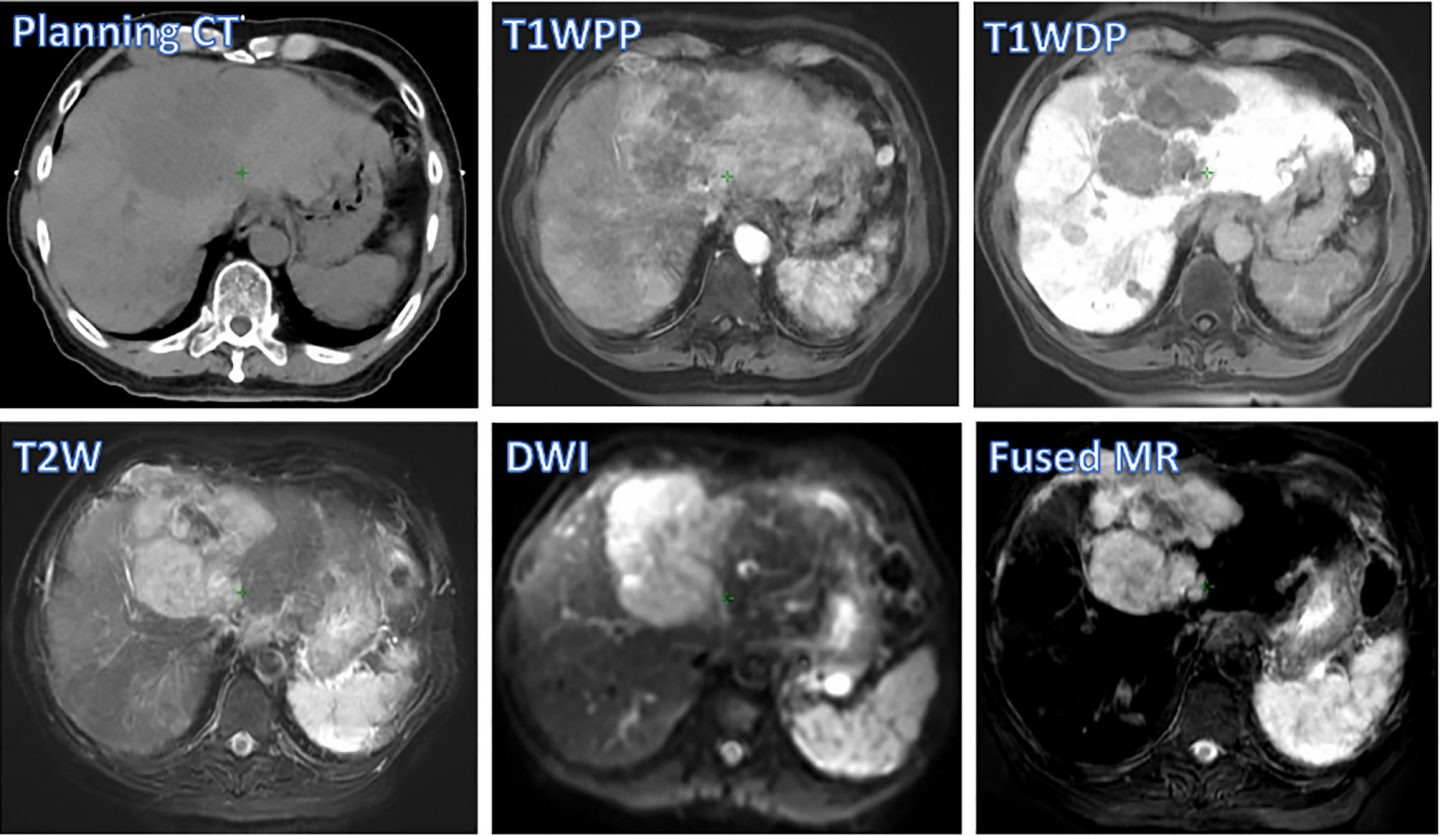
Tumor visibility of a representative patient on various images: planning CT, four original MR sets (T1W_PP_, T1W_DP_, T2W, and DWI), and fused MRI. T1W_PP_, T1-weighted MRI at portal-venous phase; T1W_DP_, T1-weighted MRI at 19-min delayed phase; T2W, T2-weighted; DWI, diffusion-weighted MRI.
